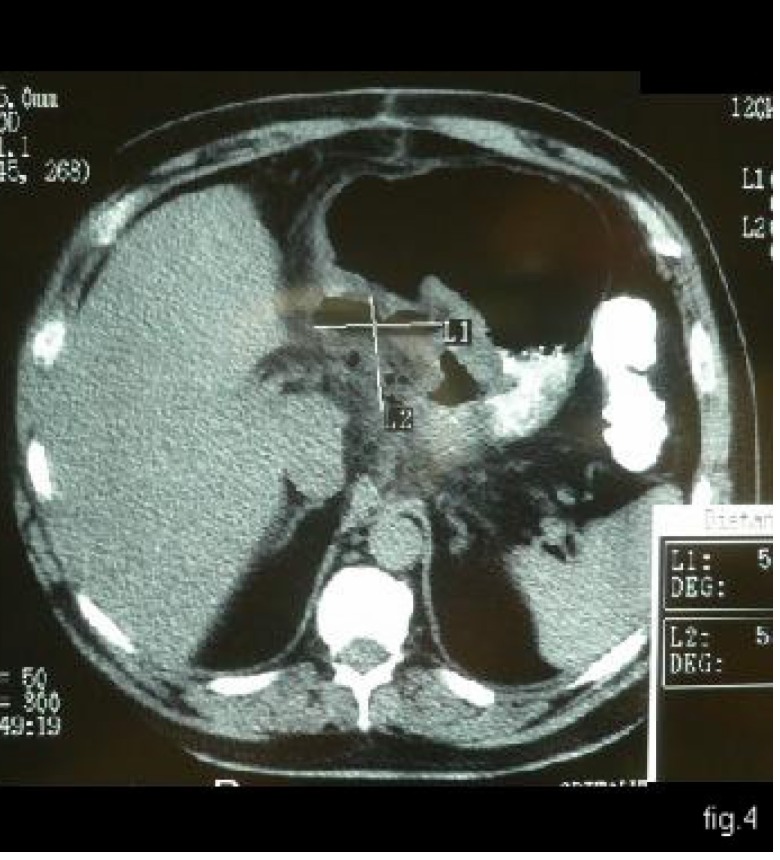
CT – collection with air-fluid level on the little gastric curve
